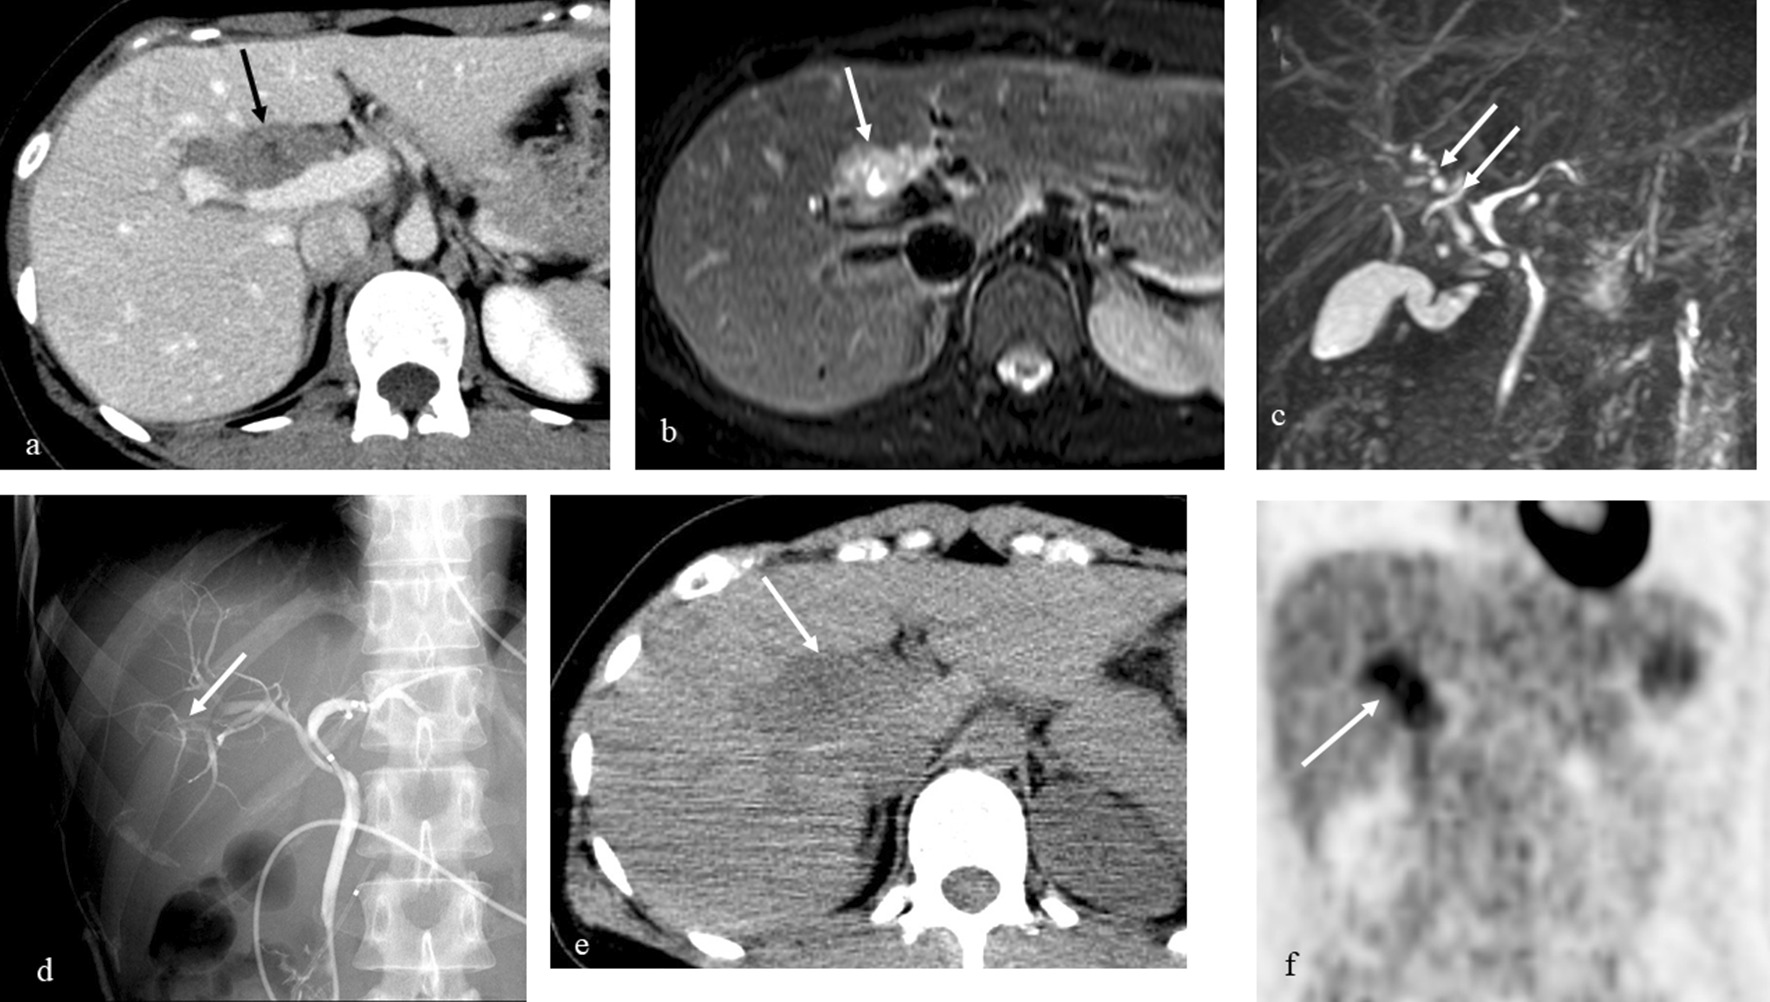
a Contrast-enhanced CT shows a clavate-shaped periportal tumor with inhomogeneous moderate enhancement (arrow). b T2-weighted imaging shows the tumor isointense to fat with cystic degeneration (arrow). c MRCP shows bile duct compression without any biliary dilatation (arrows). d ERCP also shows bile duct compression without any biliary obstruction (arrow). e The tumor has not changed at the 2nd CT (arrow). f PET shows FDG uptake of the tumor (arrow, SUV_max_ 5.0). SUV_max_ is increased slightly to 6.5 in the late phase
